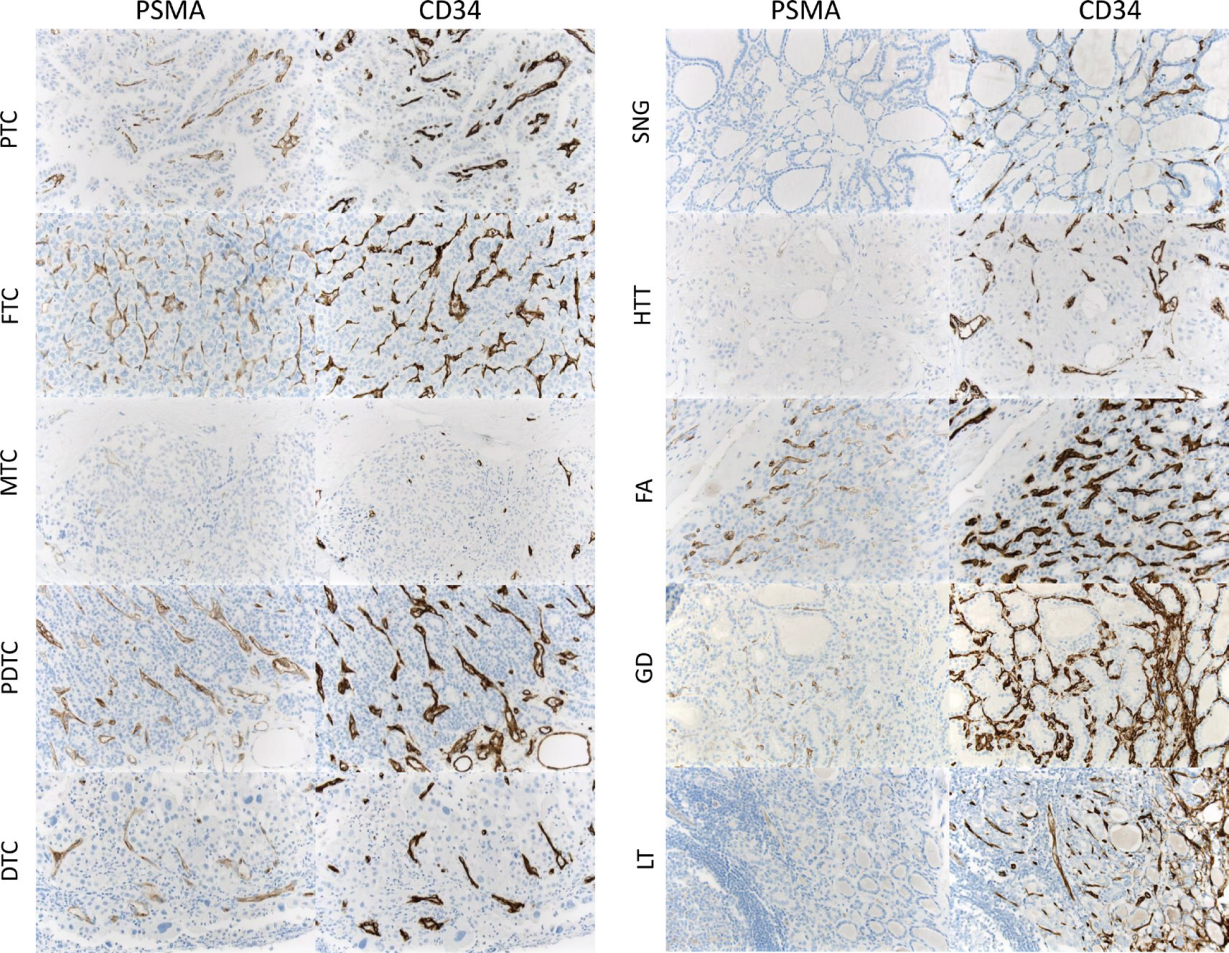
PSMA expression in malignant tumors and benign thyroid diseases (Neo-)vascular PSMA expression in different thyroid cancer subtypes and benign thyroid disease (PTC, papillary thyroid cancer; FTC: follicular thyroid cancer; MTC, medullary thyroid cancer; PDTC, poorly differentiated thyroid cancer; DTC, dedifferentiated (anaplastic) thyroid cancer; SNG, sporadic nodular goiter; FA, follicular adenoma; HTT, hyalinising trabecular thyroid tumor; GD, Grave´s disease; LT, lymphocytic thyroiditis). Vasculature was identified by means of CD31 and CD34 coexpression.
